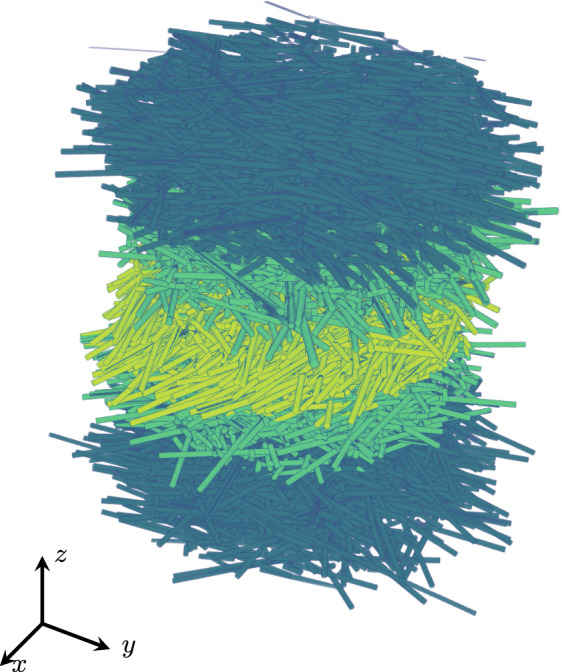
3D $$\upmu $$-CT image of an SFRP [8]
